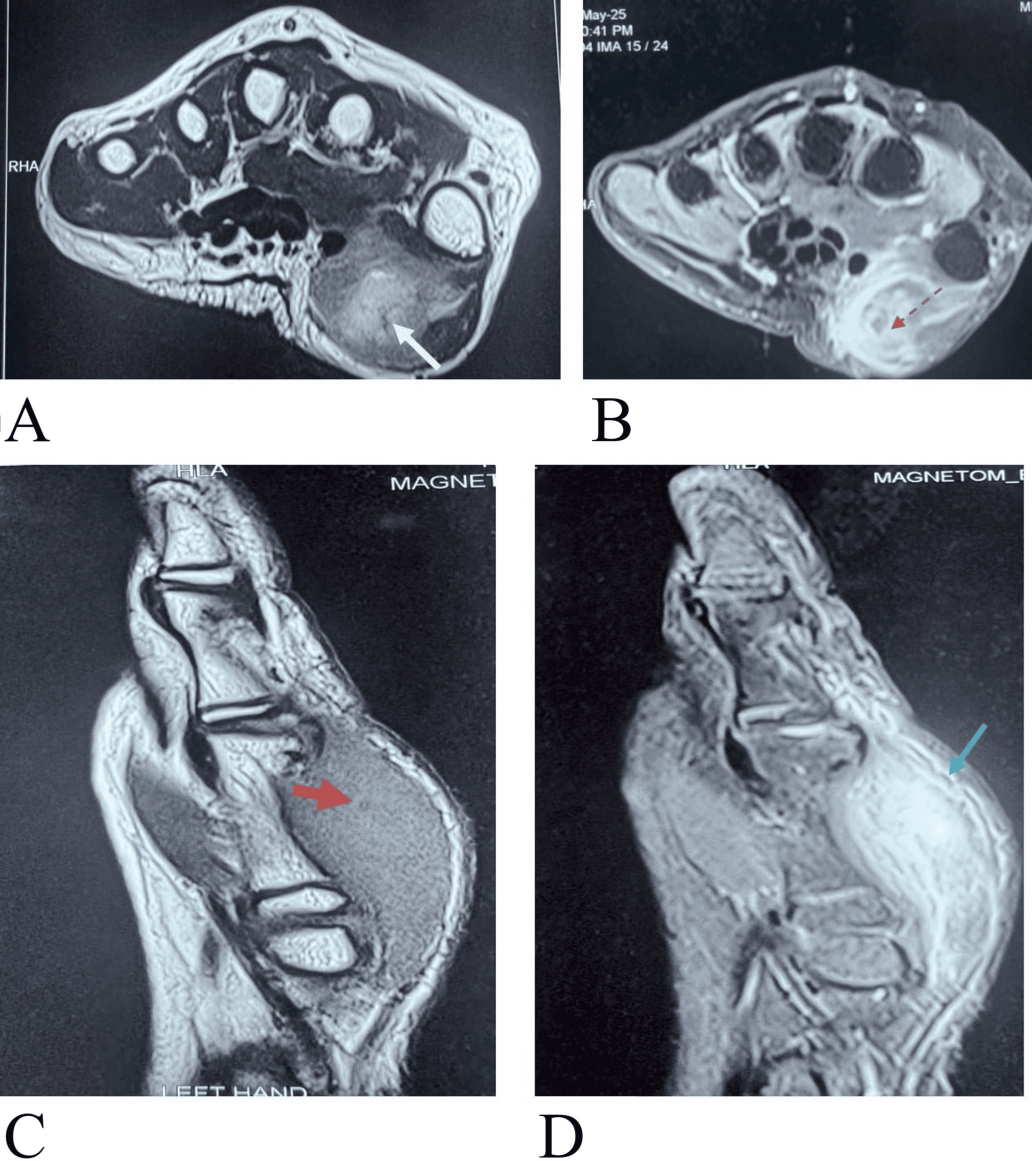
Left thenar eminence A) MRI of left hand, T2-weighted image (white arrow) shows heterogeneous cystic lesion in left thenar muscles with surrounding edema. B) T1-weighted post-contrast image (red dotted arrow) shows hyperintense lesion with marked surrounding edema. C) T1-weighted image shows hypointense lesion with hyperintense central dot suggestive of scolex (red thick arrow) with bulky left thenar muscles. D) MRI image shows bulky muscles (blue arrow) with intense surrounding edema.
